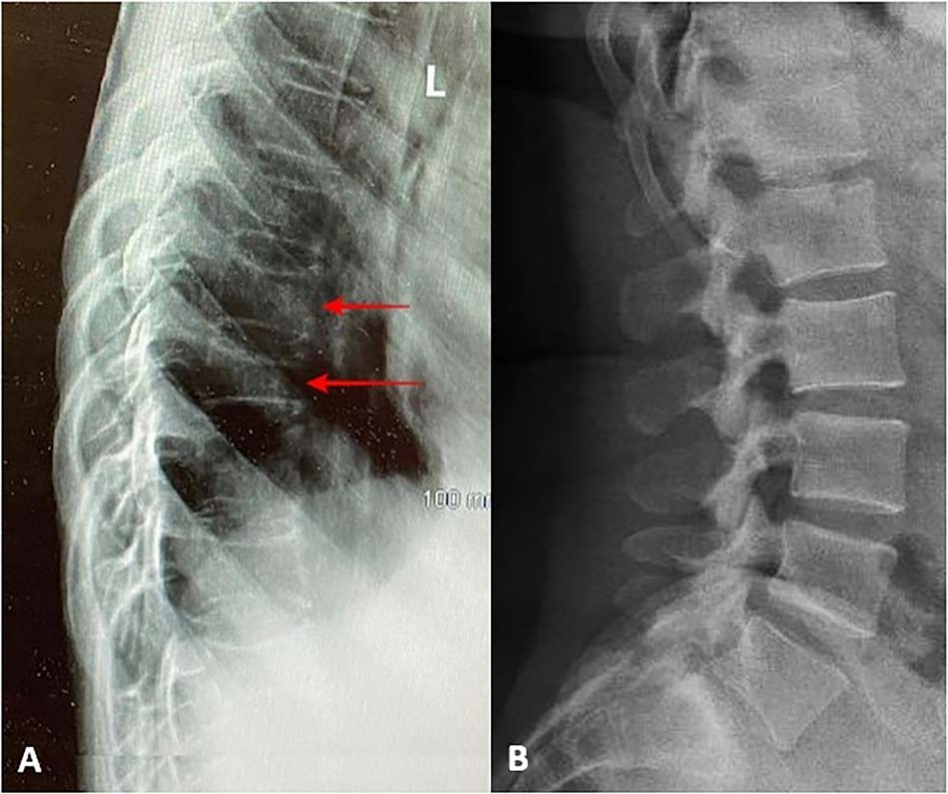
(A) Widespread biconcave and plate depression over thoracic and lumbar vertebrae (red arrows) in comparison with (B) normal thoracolumbar lateral X-ray.
